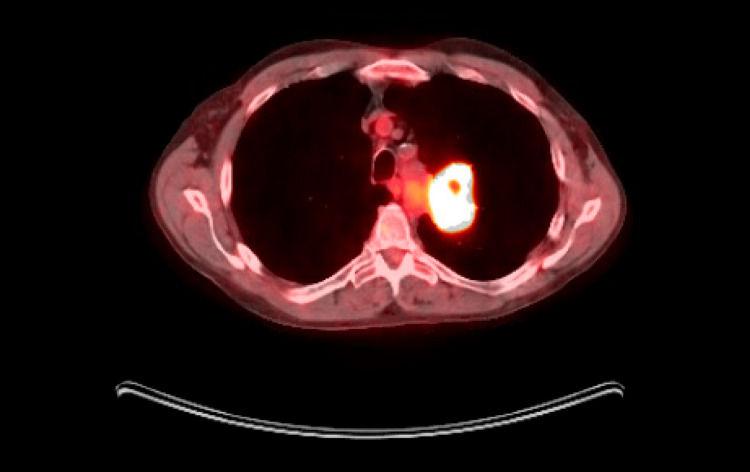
Severe FDG avidity involving a 5 x 3 cm left upper lobe mass in the supra-hilar area abutting the mediastinum, consistent with neoplasia FDG: fluorodeoxyglucose
